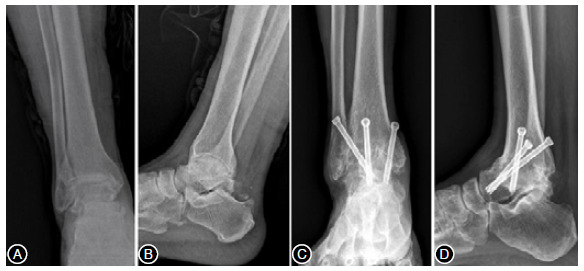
Preoperative weightbearing radiographs of the ankle. Takakura stage 4. (A) anteroposterior X-ray and (B) lateral X-ray. Anterior approach was performed with three 6.5-mm cannulated screws. Ankle anteroposterior (C) and lateral (D) radiographs view showing fusion of the ankle joint at the final follow up (13 months after operation).
